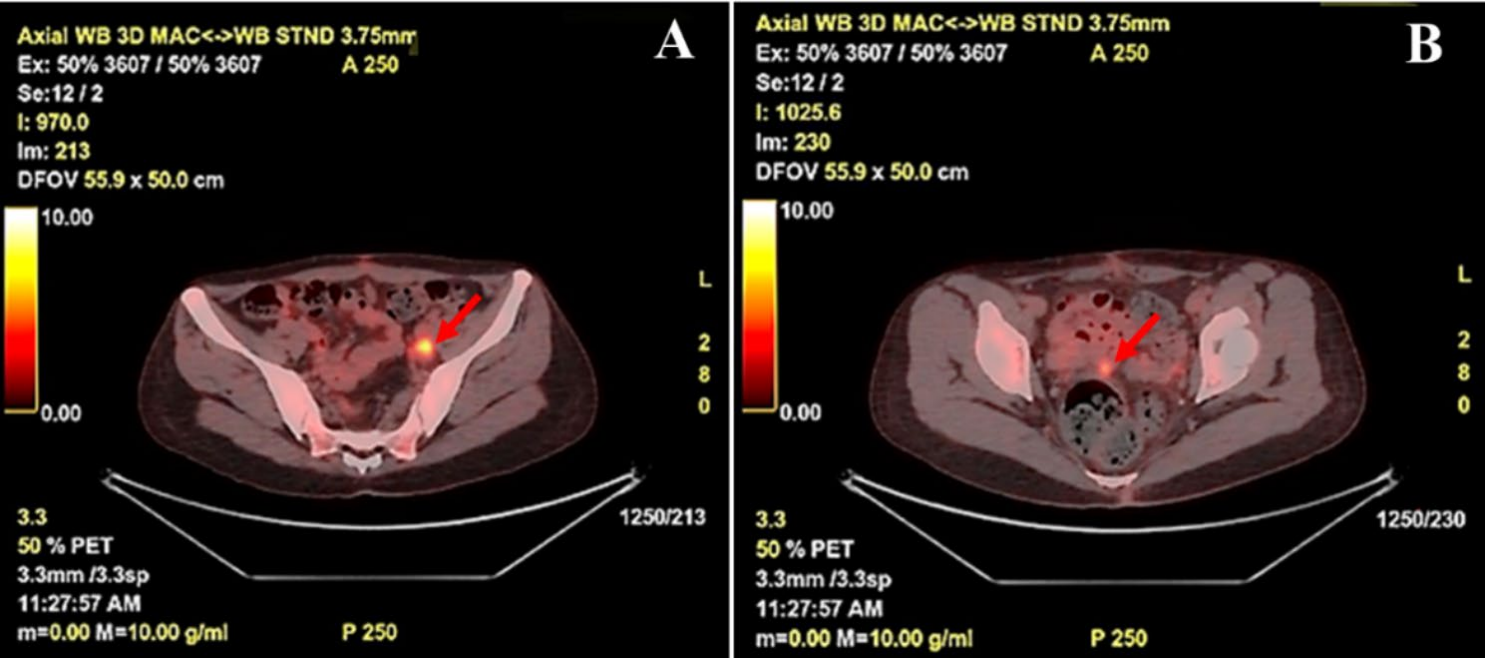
PET-CT showed hypermetabolic foci in the left iliac fossa (A) and pelvis(B)
